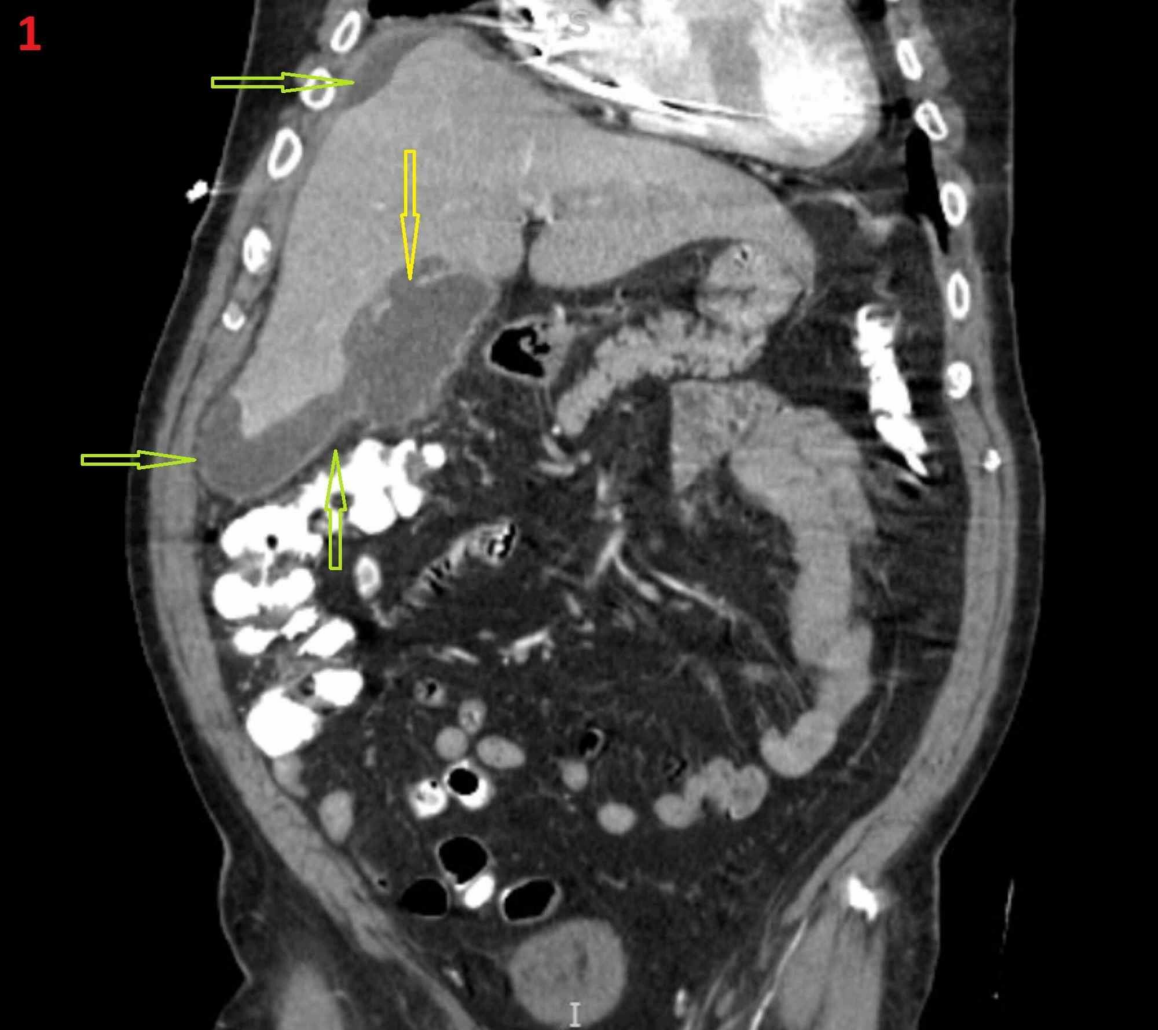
Coronal abdomen and pelvis computed tomography (CT) scan. Perihepatic fluid collection (green arrows) with hepatomegaly. There is discontinuity of the gallbladder wall (yellow arrow).
